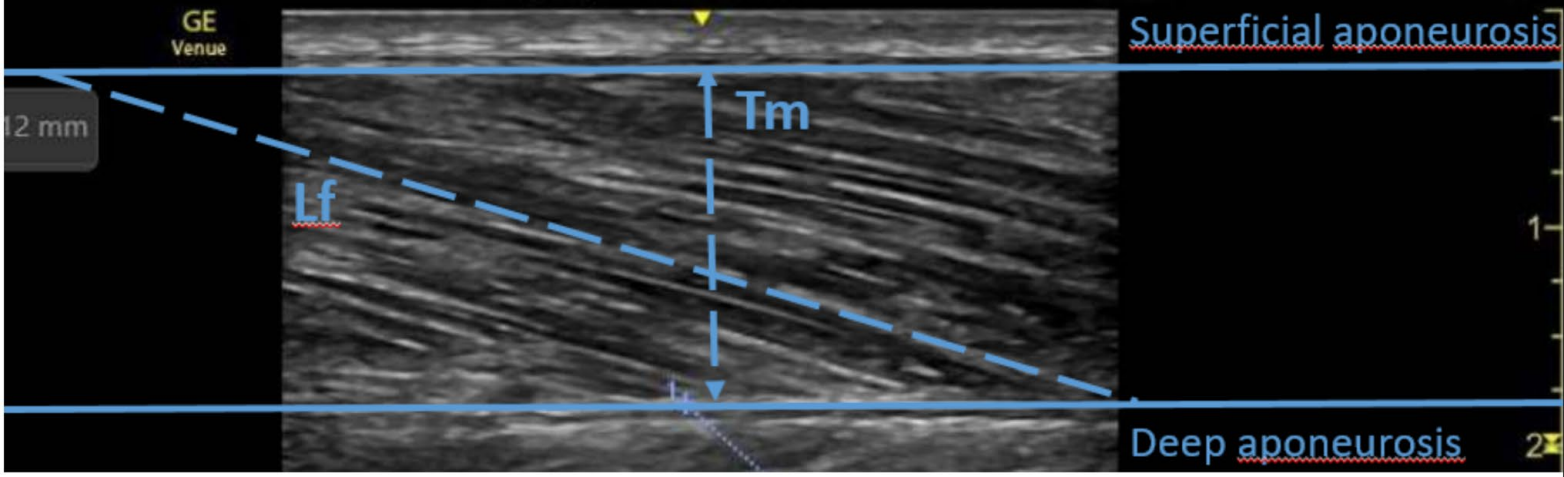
Longitudinal ultrasound image of the muscle vastus lateralis (VL), showing muscle thickness (Tm) and fascicle length (Lf)
